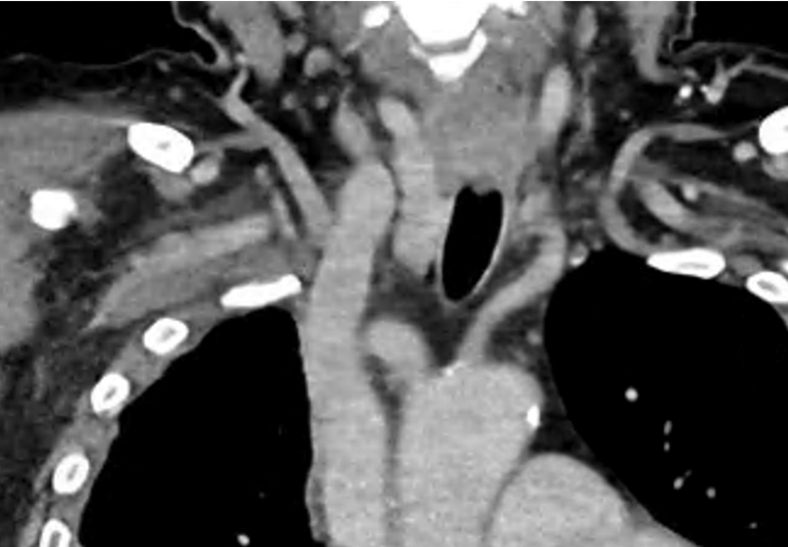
Follow-up computed tomography postoperatively. No residual tumor is visible.
